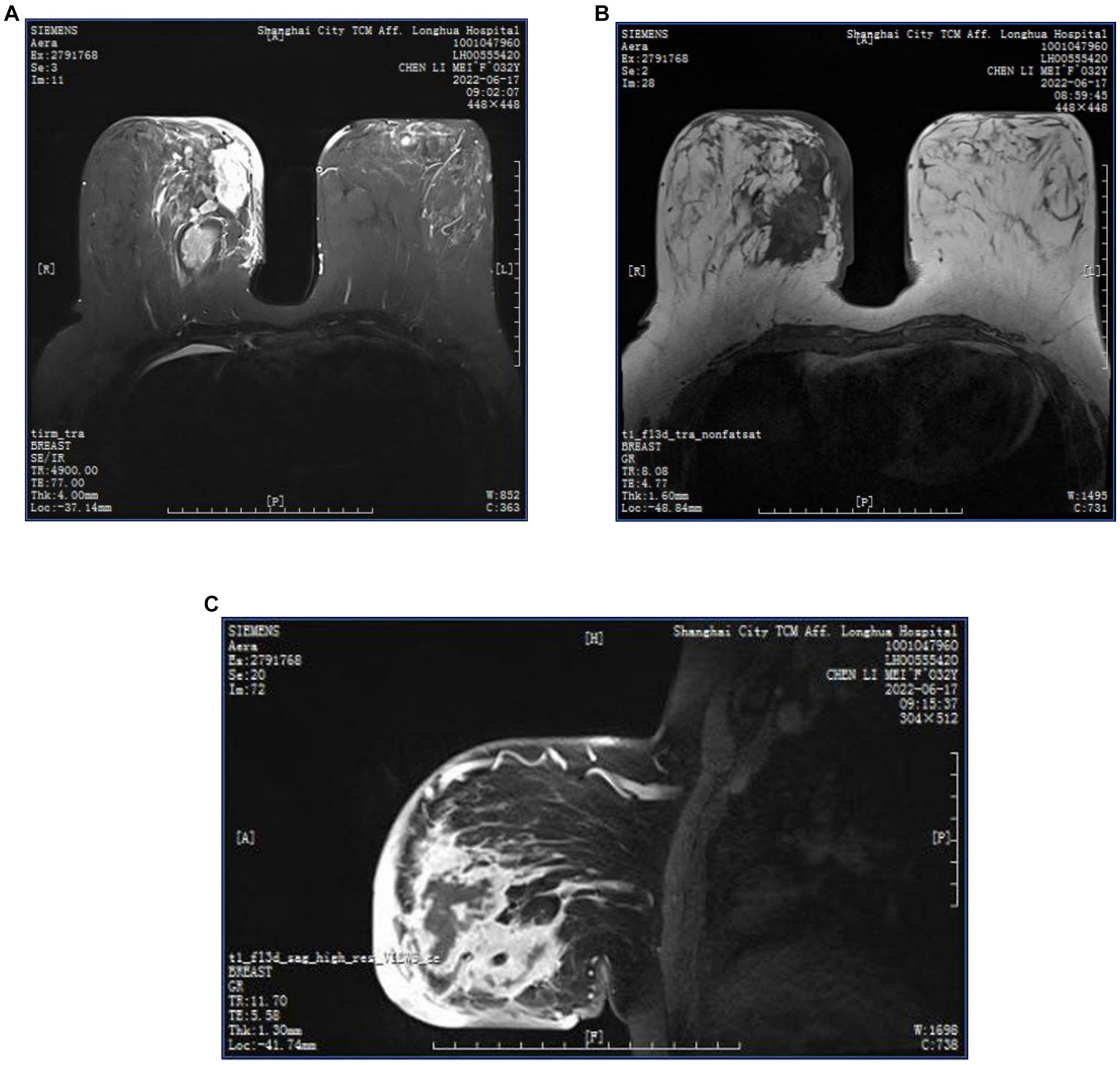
(A–C) MRI images of a GLM patient, multiple regionally distributed non-mass enhancing foci in the outer upper and inner upper quadrant of the left breast, inner upper and inner lower quadrant of the right breast, T1WI iso-slightly high signal, T2WI high signal, oedema around the foci, clusters of ring enhancement, thickening, and enhancement of the skin of the right inner breast, subcutaneous oedema. Multiple axillary lymph nodes on both sides, partially enlarged, with visible lymphatic gates.
